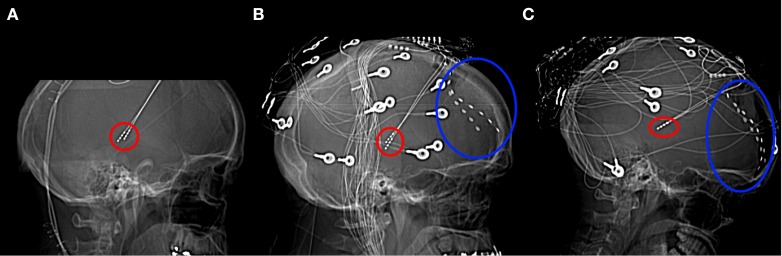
Lateral x-radiographs from Patients 1 (A), 2 (B), and 3 (C). Thalamic stimulator contacts are circled in red, subdural electrodes circled in blue. (Imaging for Patient 1 was carried out only after the removal of the subdural electrodes).
